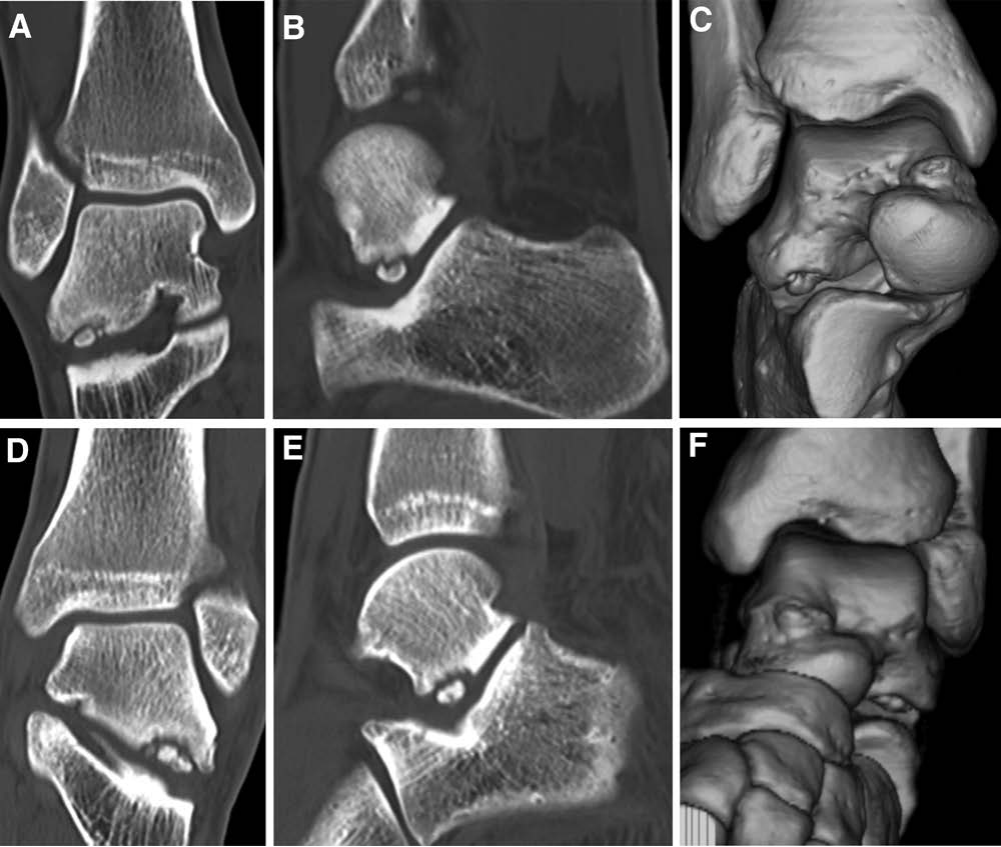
Preoperative computed tomography of the right (A–C) and the left (D–F) feet reveal bilateral osteochondral lesions on the lateral process of the talus involving the subtalar joint.
